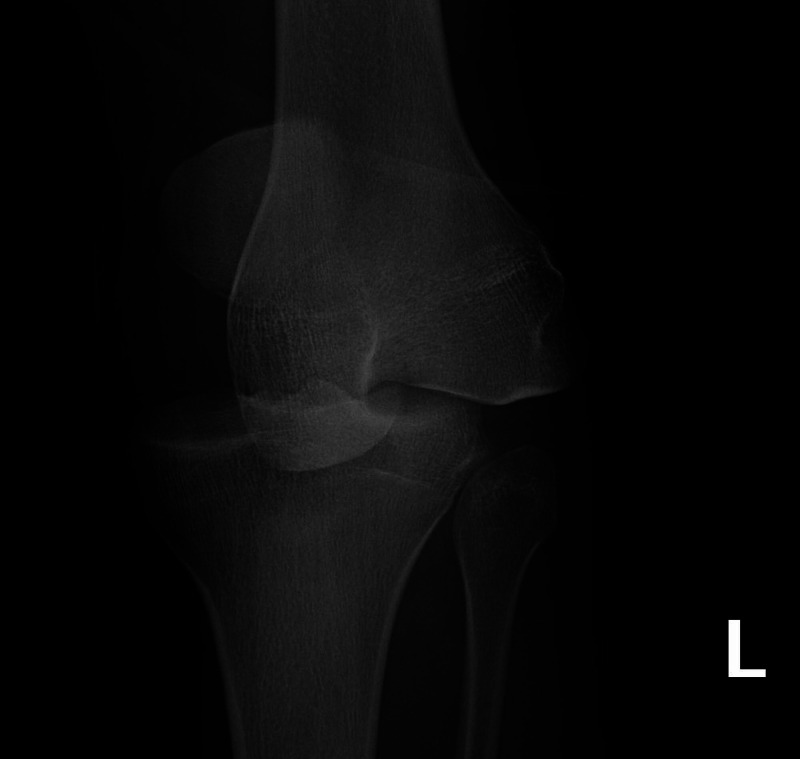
Anterior–posterior radiograph of the left knee in case 1 Anterior–posterior radiograph of the left knee demonstrating acute knee dislocation.
